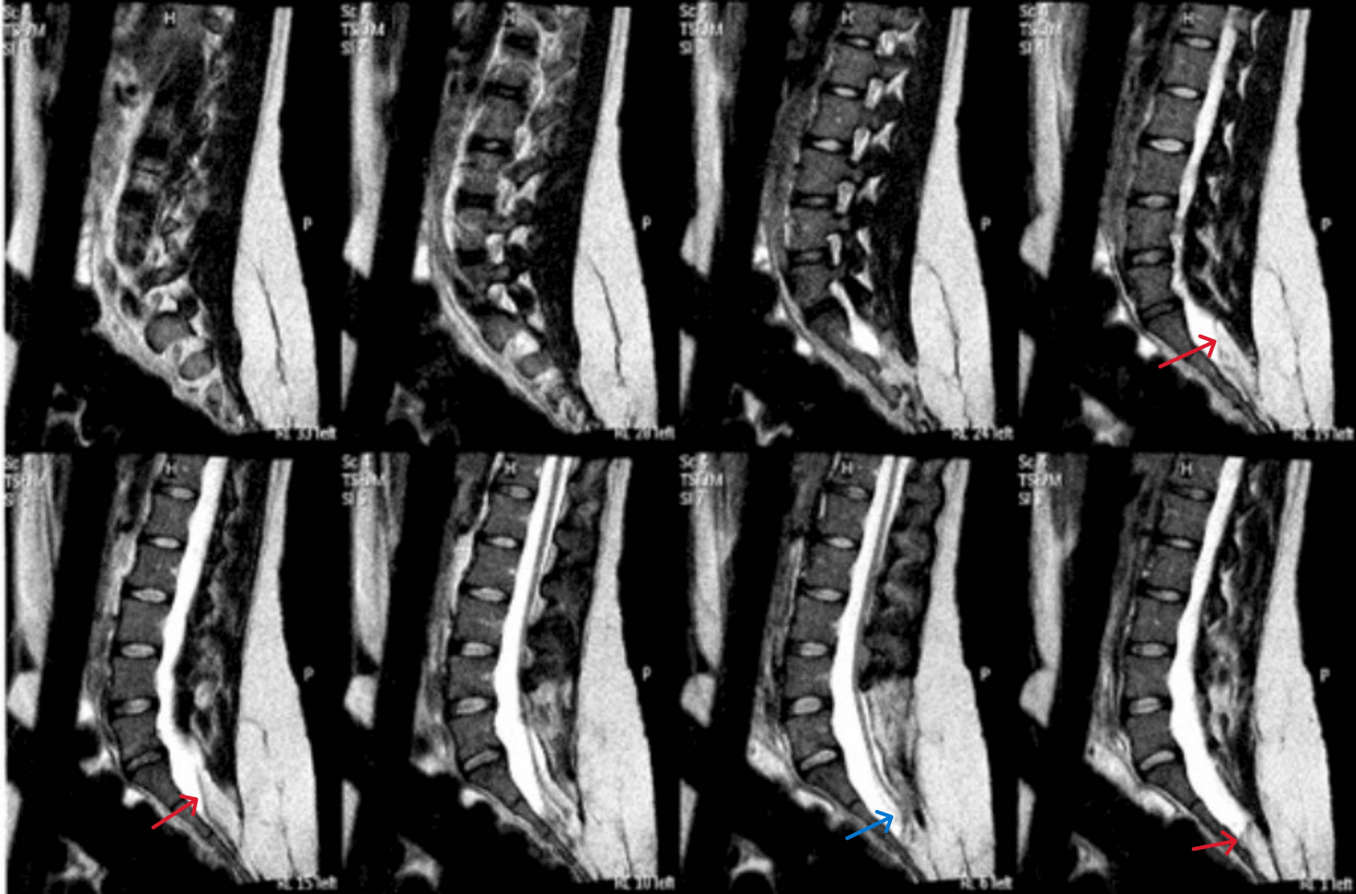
Magnetic resonance imaging (MRI) of the lumbosacral spine Sagittal T2-weighted MRI views demonstrating a complex closed spinal dysraphism consistent with lipomyelomeningocele (red arrow) and tethered cord (blue arrow) syndrome.
